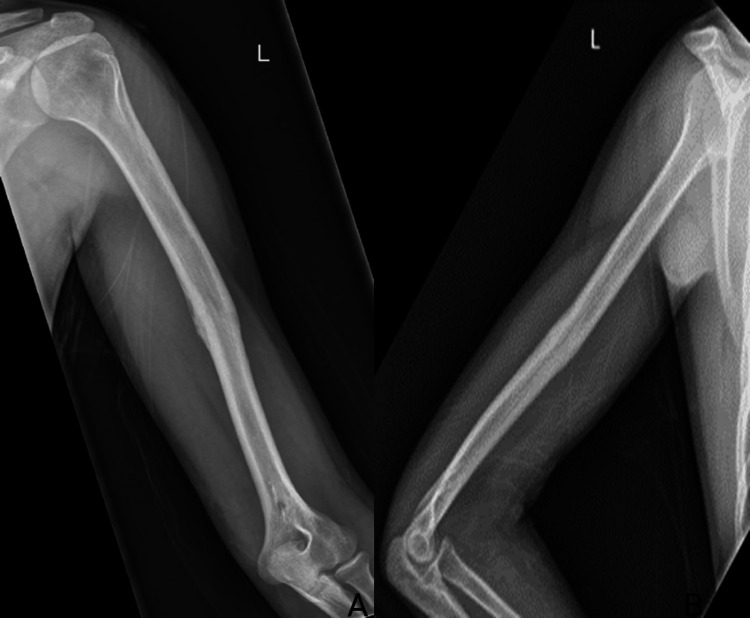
AP and lateral X-rays post removal of the K-wires. (A) AP view of the left humerus. (B) Lateral view of the left humerus. AP, antero-posterior; K-wires, Kirschner wires
